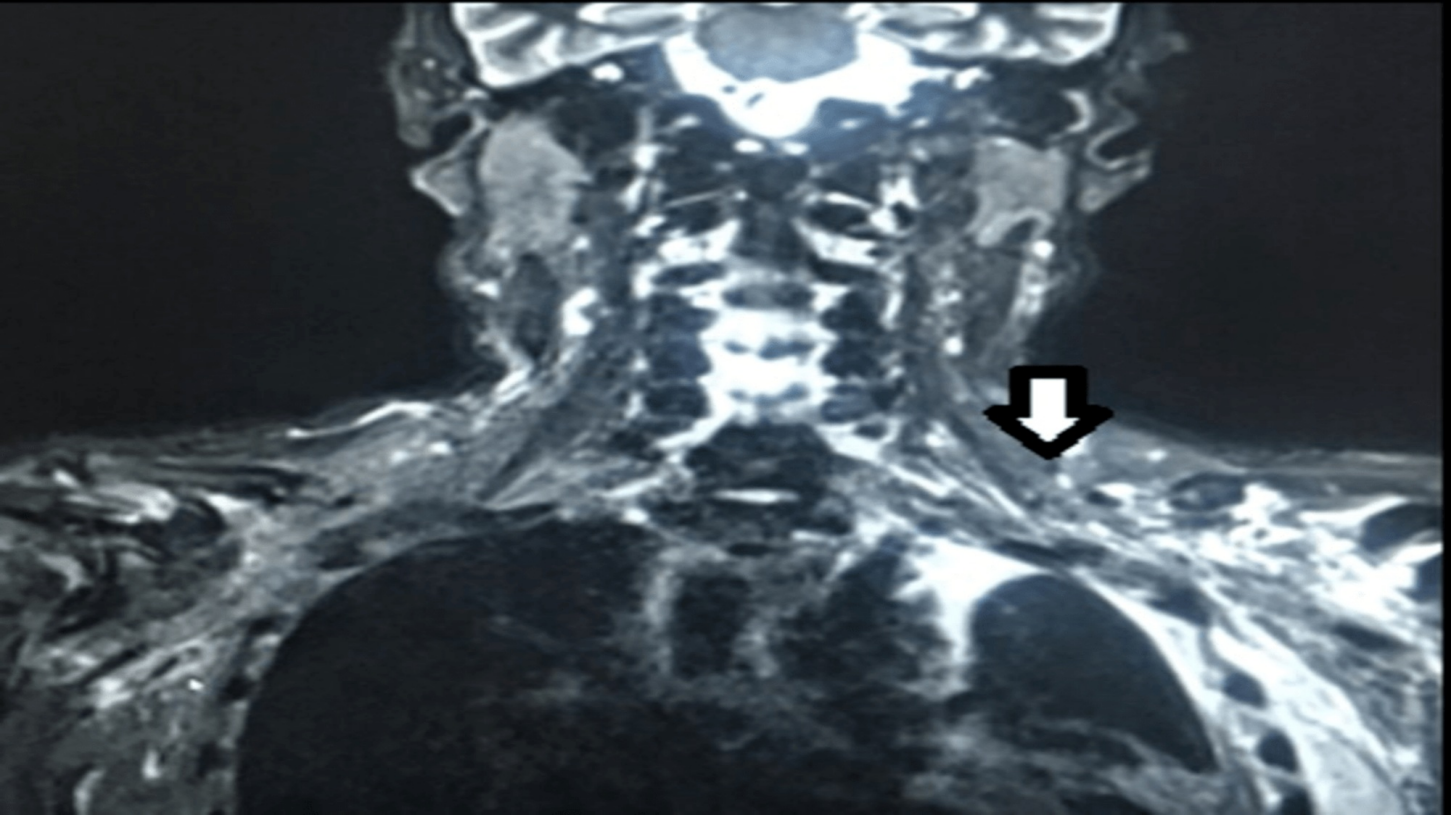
T2-weighted coronal view showing inflammatory process invading chest wall and surrounding left brachial plexus cords in left infra-scapular and axillary region
